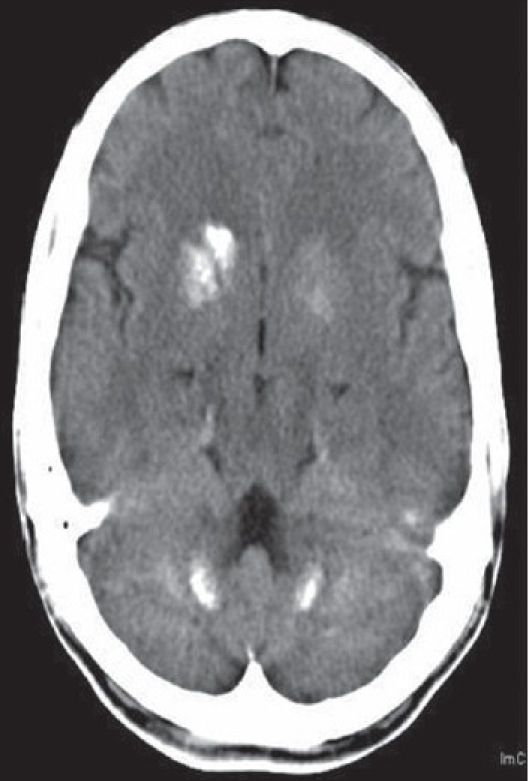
Plain CT head shows bilateral calcification in the anterior lentiform nucleus, caudate nucleus, and dentate nucleus of cerebellum
